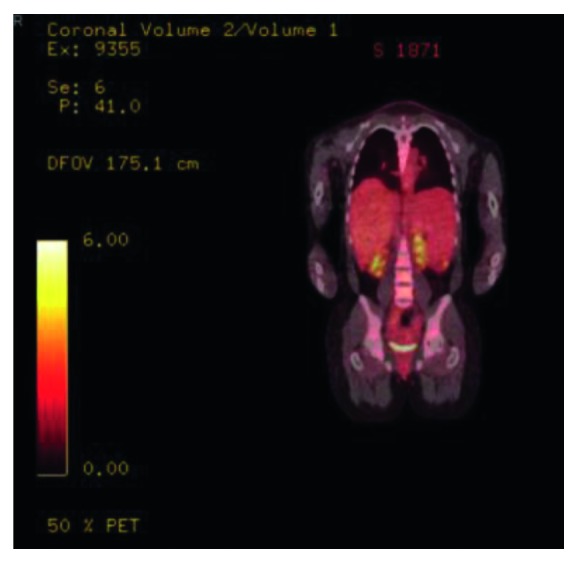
PET scan, initial coronal section at presentation, showing marked splenomegaly. The spleen measured approximately 20 cm in length with relatively focal areas of increased radiotracer uptake in the splenic parenchyma with max SUV 4.4, compared to background max SUV throughout the spleen ranging 2.5–3.0. Background radiotracer uptake throughout the liver was very slightly greater than of the spleen, 2.7–3.2. There was also a focus of asymmetric increased radiotracer uptake in the left parapharyngeal soft tissues with associated soft tissue thickening on CT images and maximum SUV 3.5.
